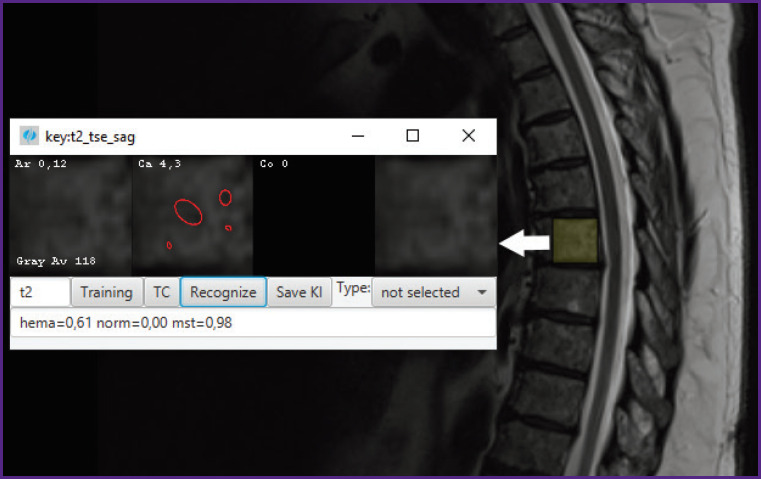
Recognition of a metastatic lesion on Th6 by sagittal T2- weighted MRI: mst probability — 0.98
